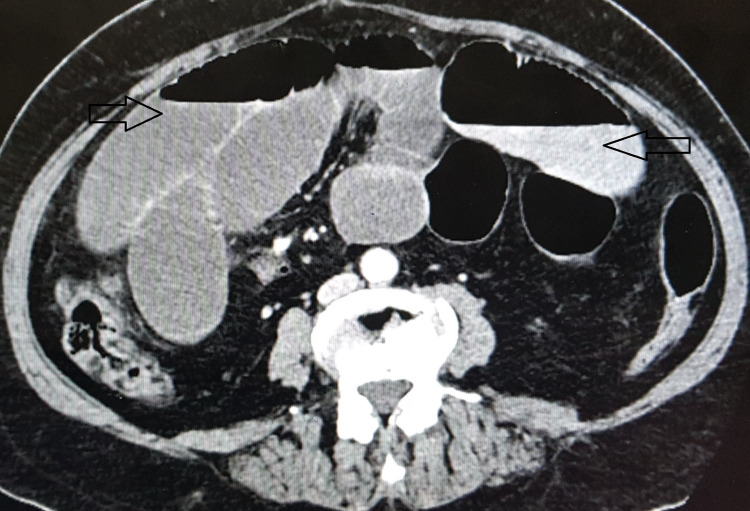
Computed tomography of the abdomen showing dilated small bowel loops with air-fluid levels (arrows).
